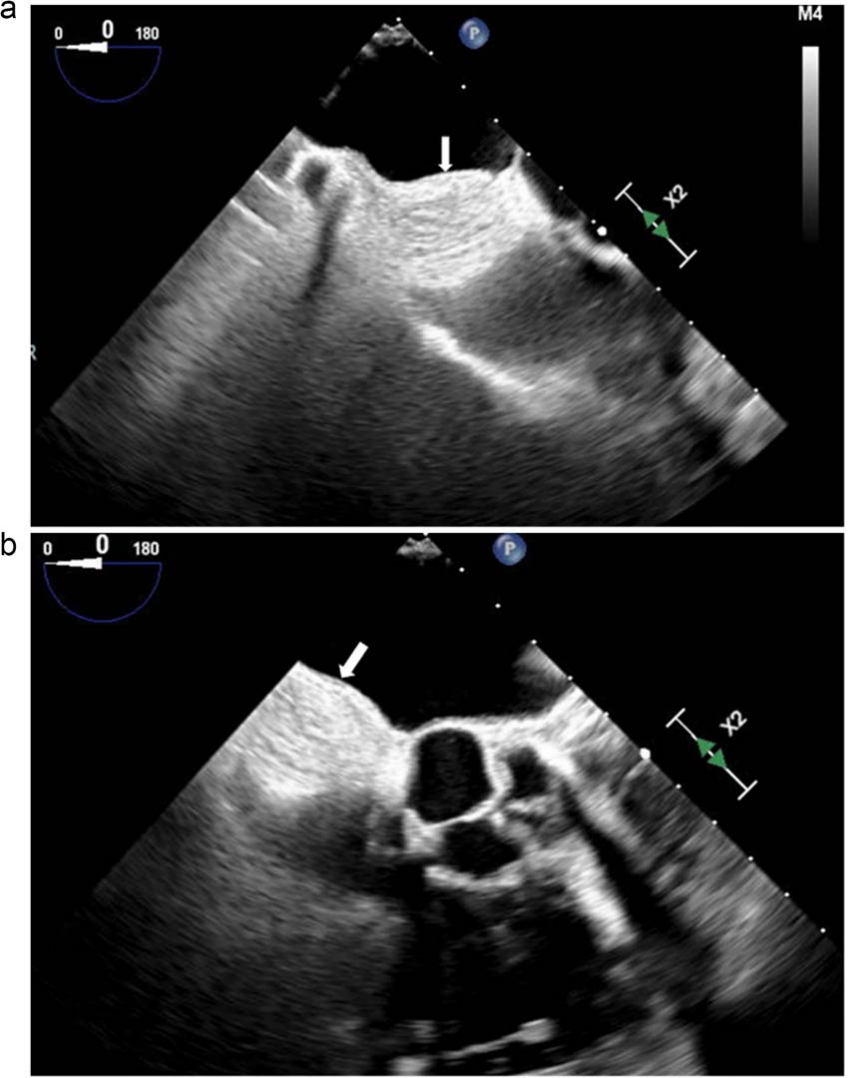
(a and b) Transesophageal echocardiogram. Arrow denotes mass involving the interatrial septum.
